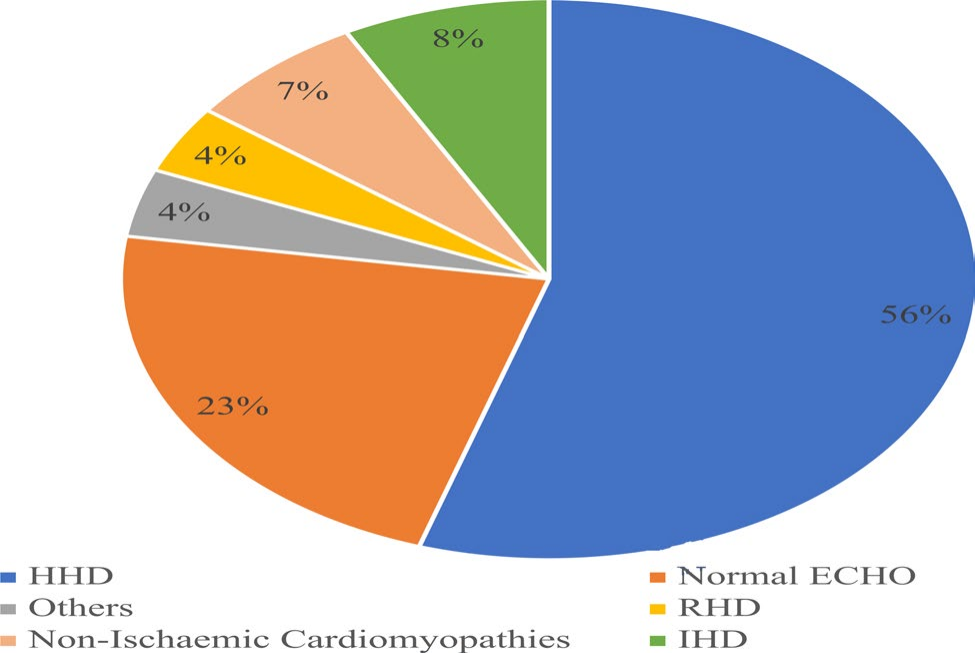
Pattern of echocardiographic diagnosis (N = 1050) *HHD*: *Hypertensive heart disease*, *IHD*: *Ischaemic heart disease*, *RHD*: *Rheumatic heart disease.OTHERS*: *Mitral valve prolapse (1.4%), Cor-pulmonale (1%), Degenerative Valvular heart disease (0.6%), Pericardial diseases (0.6%), Adult congenital heart diseases (0.6%-Congenitally corrected transposition of great arteries, ventricular septal defect, atrial septal defect with partial anomalous pulmonary venous return and tetralogy of fallot), Left atrial myxoma (0.2%) and myocarditis (0.2%).*
